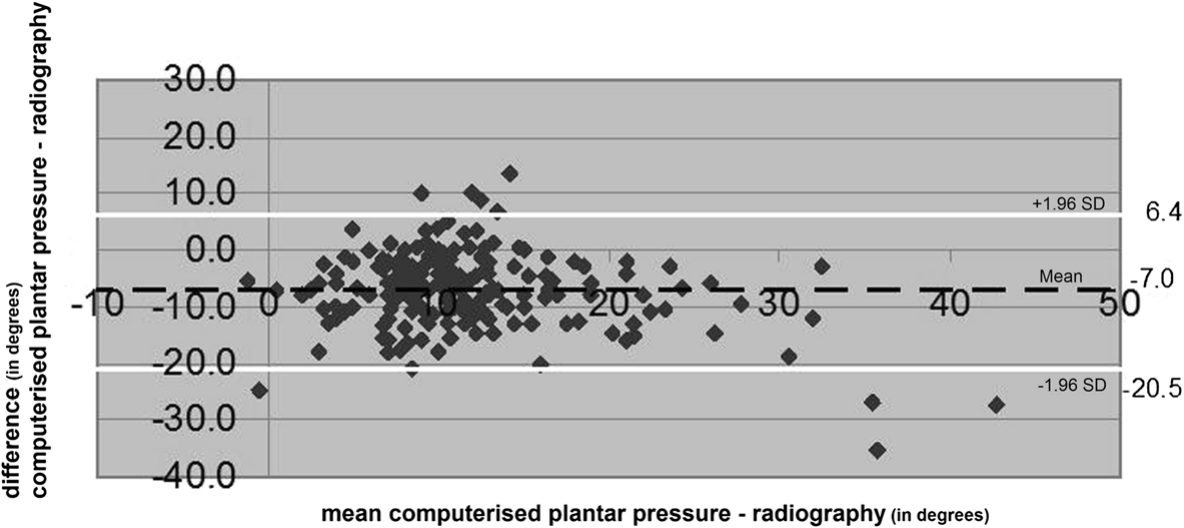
The range of the difference between the results of computerised plantar pressure measurement of HVA and radiographic measurement of HVA. (Degrees). The mean difference (black discontinuous line) and the prediction interval (white lines) are presented.
